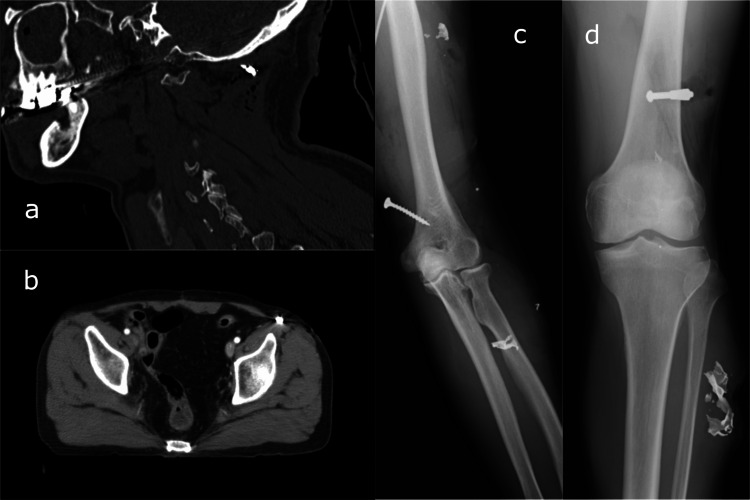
Computed tomography scan and radiograph of the patient. Foreign bodies such as nails and metal fragments were found in the neck (a), trunk (b), left upper limb (c), left lower limb (d).
